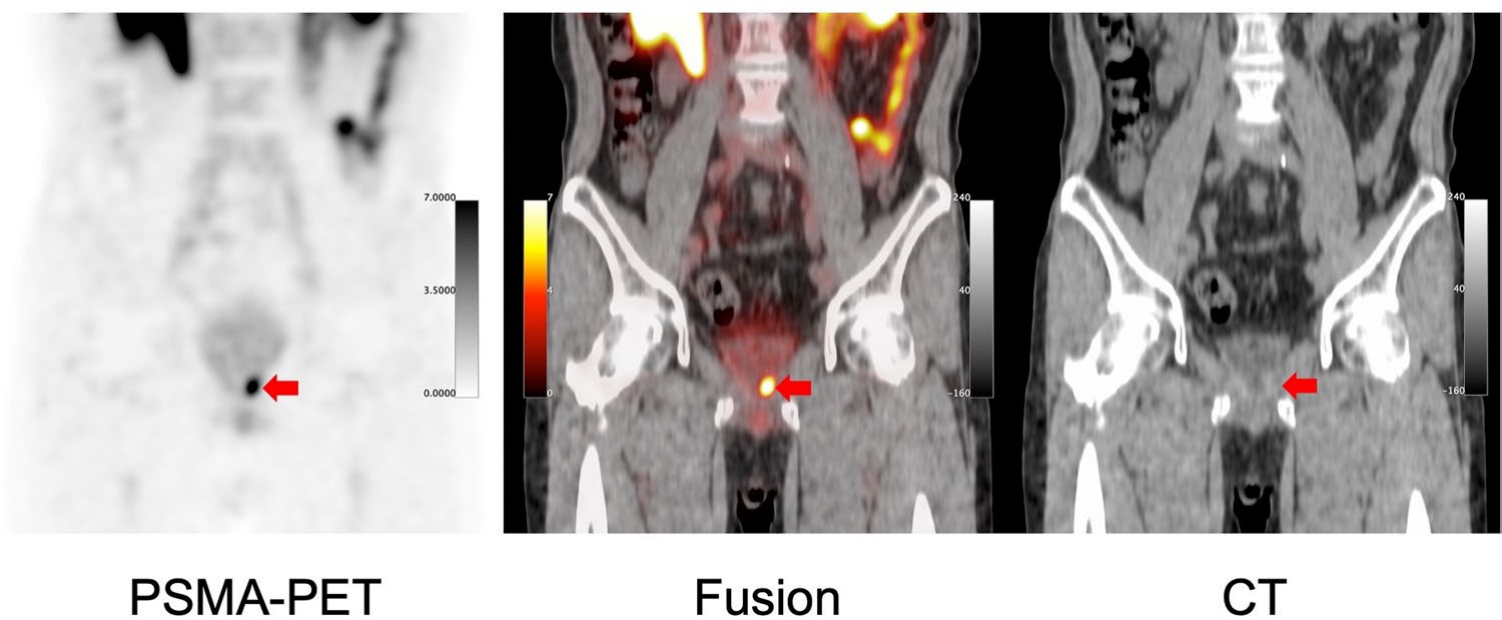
BCR patient after radical prostatectomy. Focal uptakes (red arrows) are detected at the edge of the bladder on [^18^F]PSMA-1007 PET (PSA at PET: 0.19 ng/mL). The patient is treated by radiation therapy targeting the PSMA-positive lesion and PSA values return to normal level (< 0.01 ng/mL)
